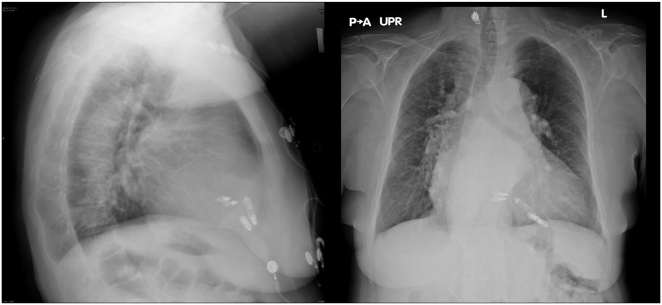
Follow-up X-rays showing parallel placement of both devices.
